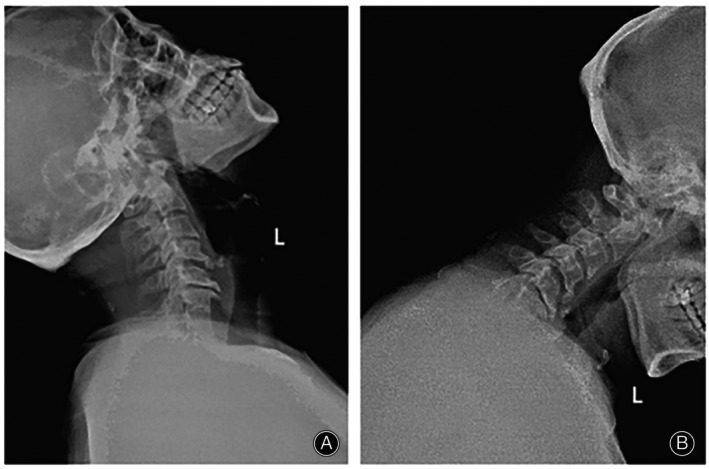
X‐ray at 24 months after the operation. The cervical spine dynamic radiograph showed that the physiological curvature of the cervical spine remained good without cervical instability (A, B).
